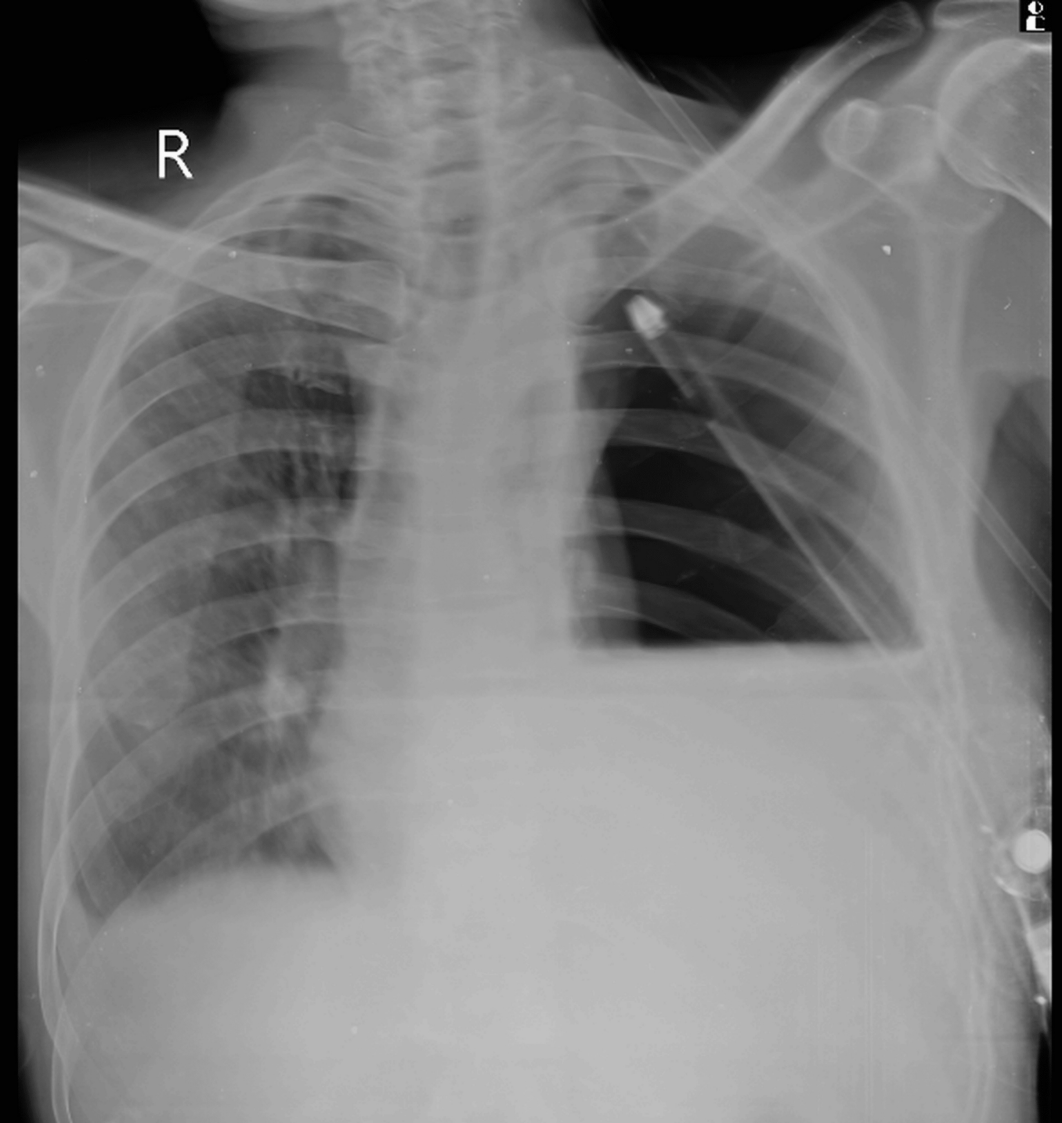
Chest X-ray suggestive of left lung collapse with hydropneumothorax with intercostal drain in situ
